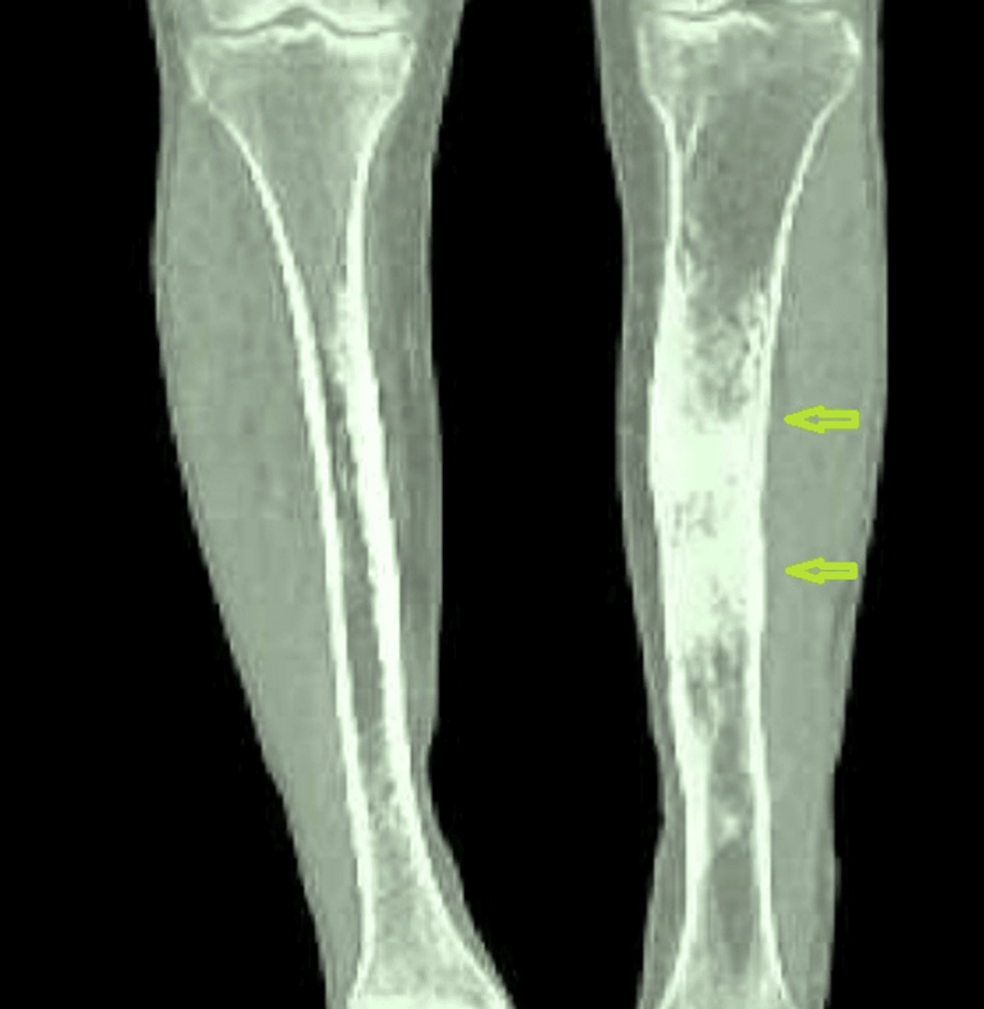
Tibial osteomyelitis CT coronal depicting periostitis and inflammation (infection) in the surrounding tissue.
